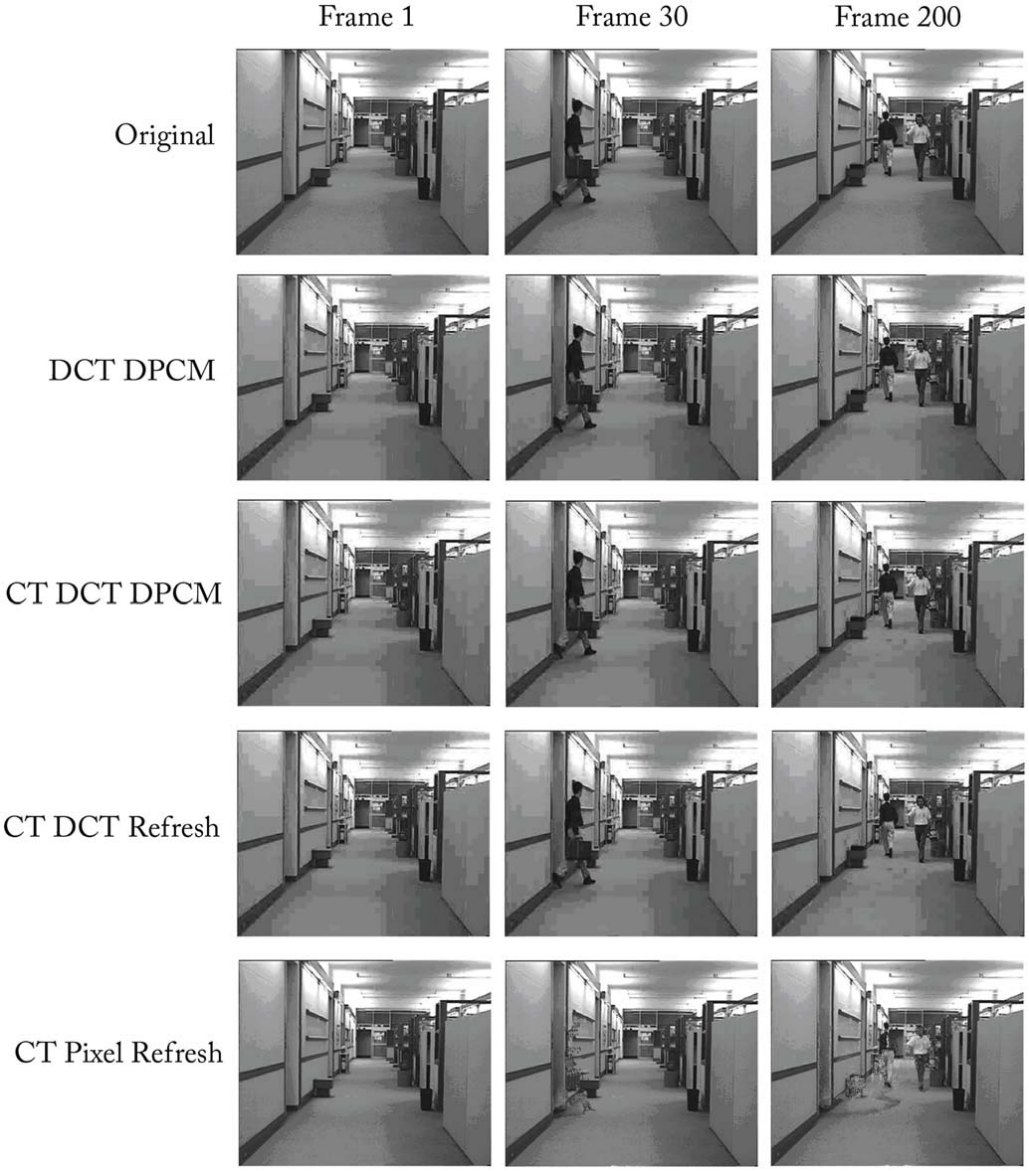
Sample frames from the original video test sequence and the compressed output from each encoder using the parameter *Q = 48*, δ = 5 and Θ = 8 for the DCT encoders and δ = 10 for the CT Pixel Refresh. Frame 1 is the start of the sequence, Frame 30 is where a man begins to enter the scene and Frame 200 is the final image in the video. All of the DCT encoders have similar compression artifacts, mainly a result of heavy DCT quantization. The CT Pixel Refresh coder does not have blocking artifacts, but missing and trailing pixels.
